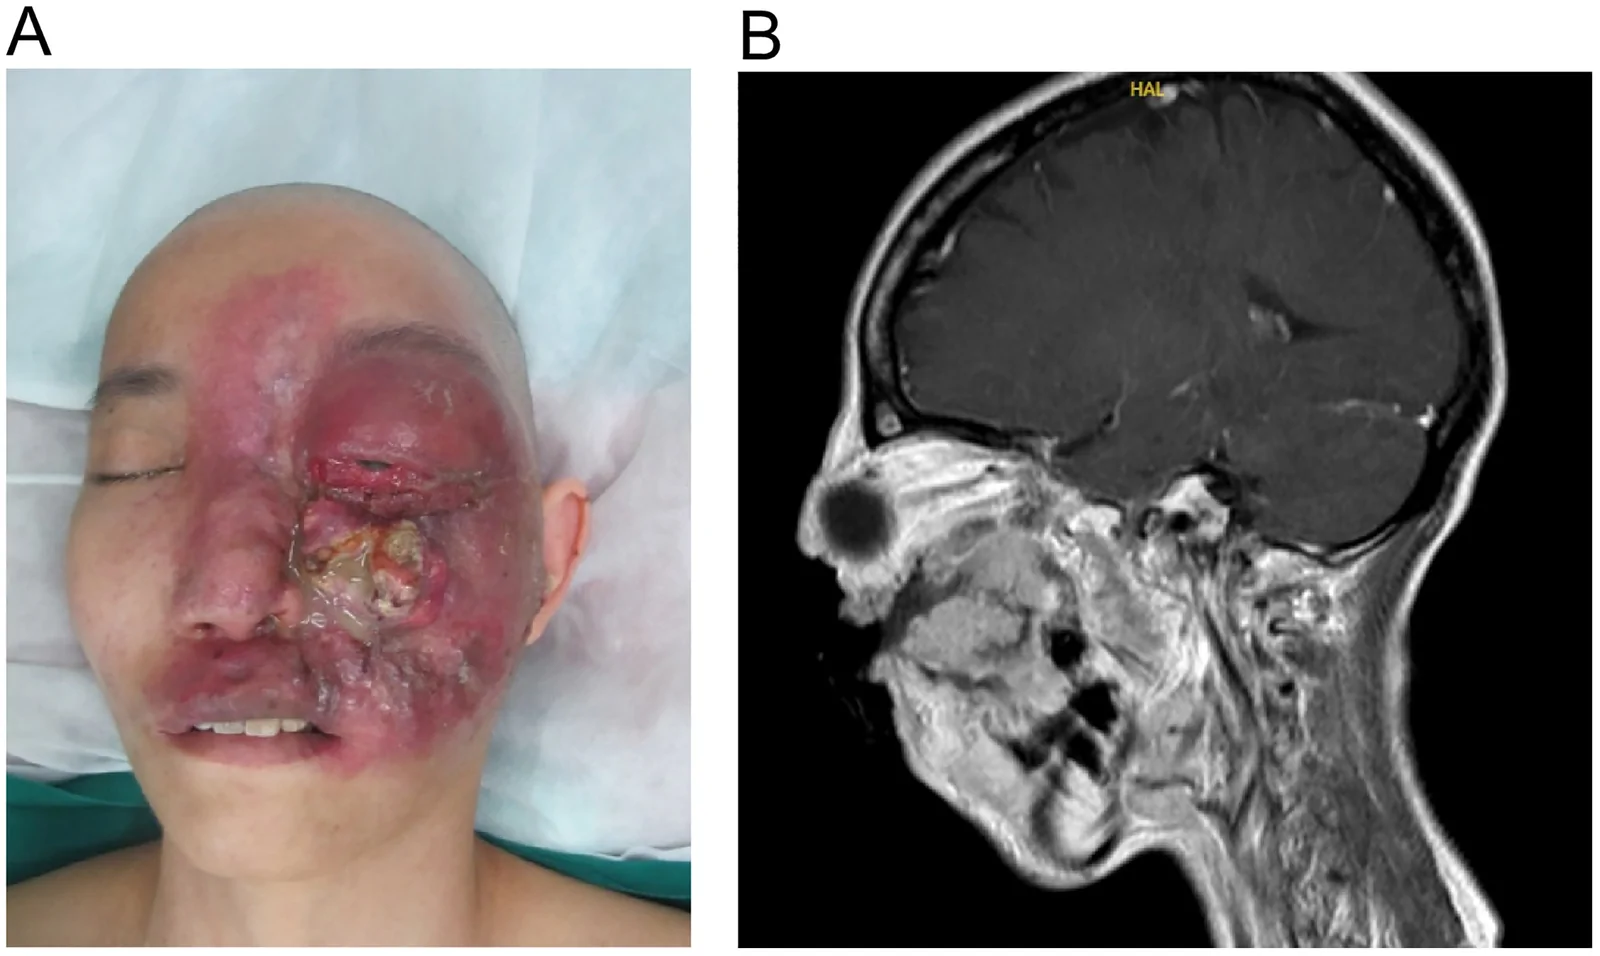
A 38-year-old woman with a Schobinger stage III/Yakes type IV AVMs associated with left maxillary squamous cell carcinoma (Case 2). A large blushing mass occupies the left craniomaxillofacial region, with tumor ulceration and active bleeding (A). Magnetic resonance imaging demonstrates a large soft-tissue mass in the left craniofacial region invading the craniofacial bones and orbit, resulting in cheek skin ulceration and tissue defects (B).
